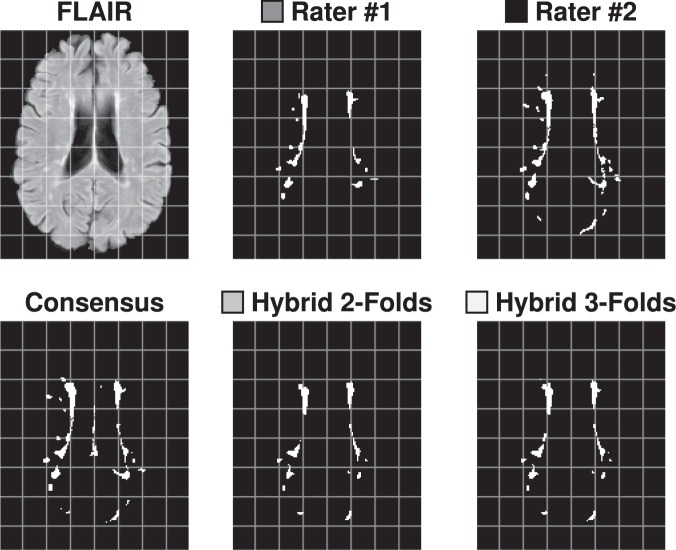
Shown on the top row are an axial slice of the FLAIR image for a subject from the Test data set, and the corresponding segmentations by Rater #1, Rater #2. On the bottom row are the corresponding slices for the Consensus Delineation (labeled Consensus) and the hybrid algorithm with 2-folds (labeled Hybrid 2-Folds) and with 3-folds (labeled Hybrid 3-Folds).
